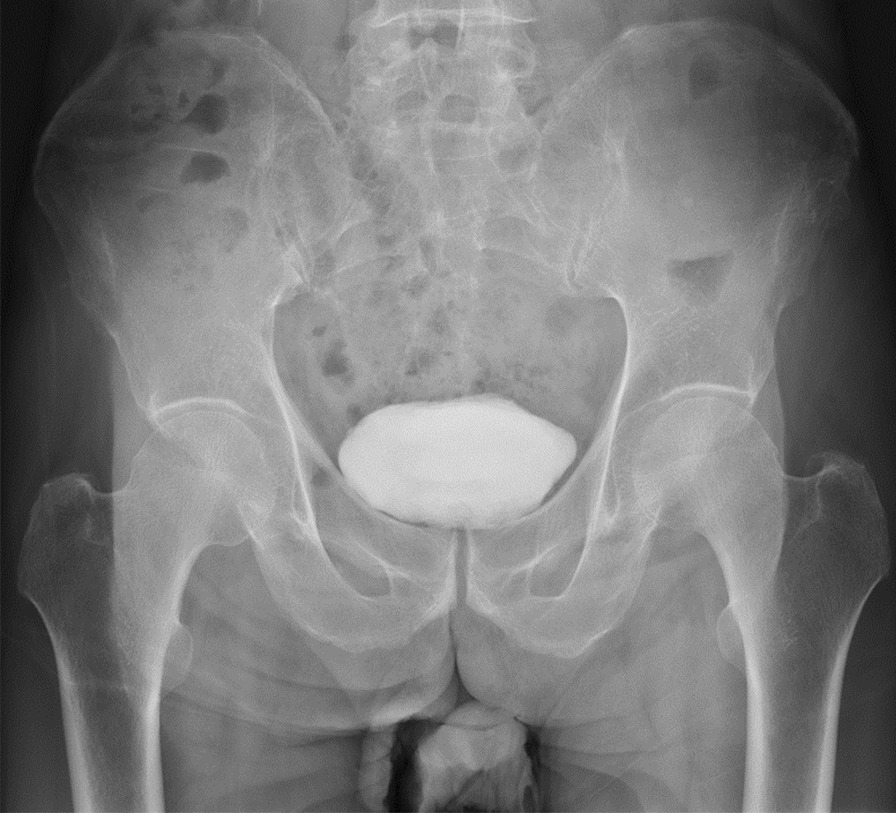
Anteroposterior pelvis radiographs taken at initial patient presentation showing no obvious abnormalities
